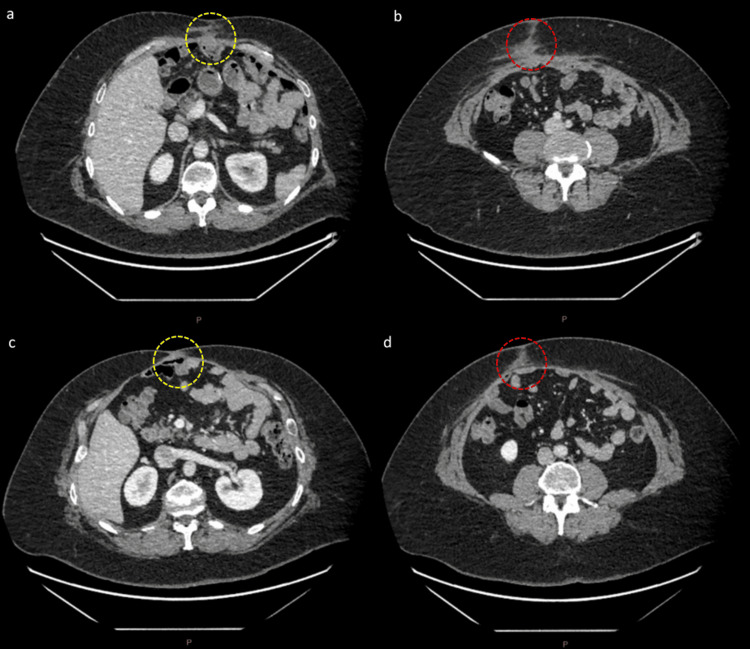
CT abdomen portal venous phase 8 months post-surgery and 1.8 years post-surgery a: Axial slice with 0.625 mm thickness, W:334/L:12, demonstrating no evidence of recurrence at the superior tumour site (yellow dotted oval). b: Axial slice with 0.625 mm thickness, W:334/L:12, demonstrating no evidence of recurrence at the inferior tumour site (red dotted oval). c: Axial slice with 0.625 mm thickness, W:400/L:40, demonstrating no evidence of recurrence at the superior tumour site (yellow dotted oval). d: Axial slice with 0.625 mm thickness, W:400/L:40, demonstrating no evidence of recurrence at the inferior tumour site (red dotted oval).
